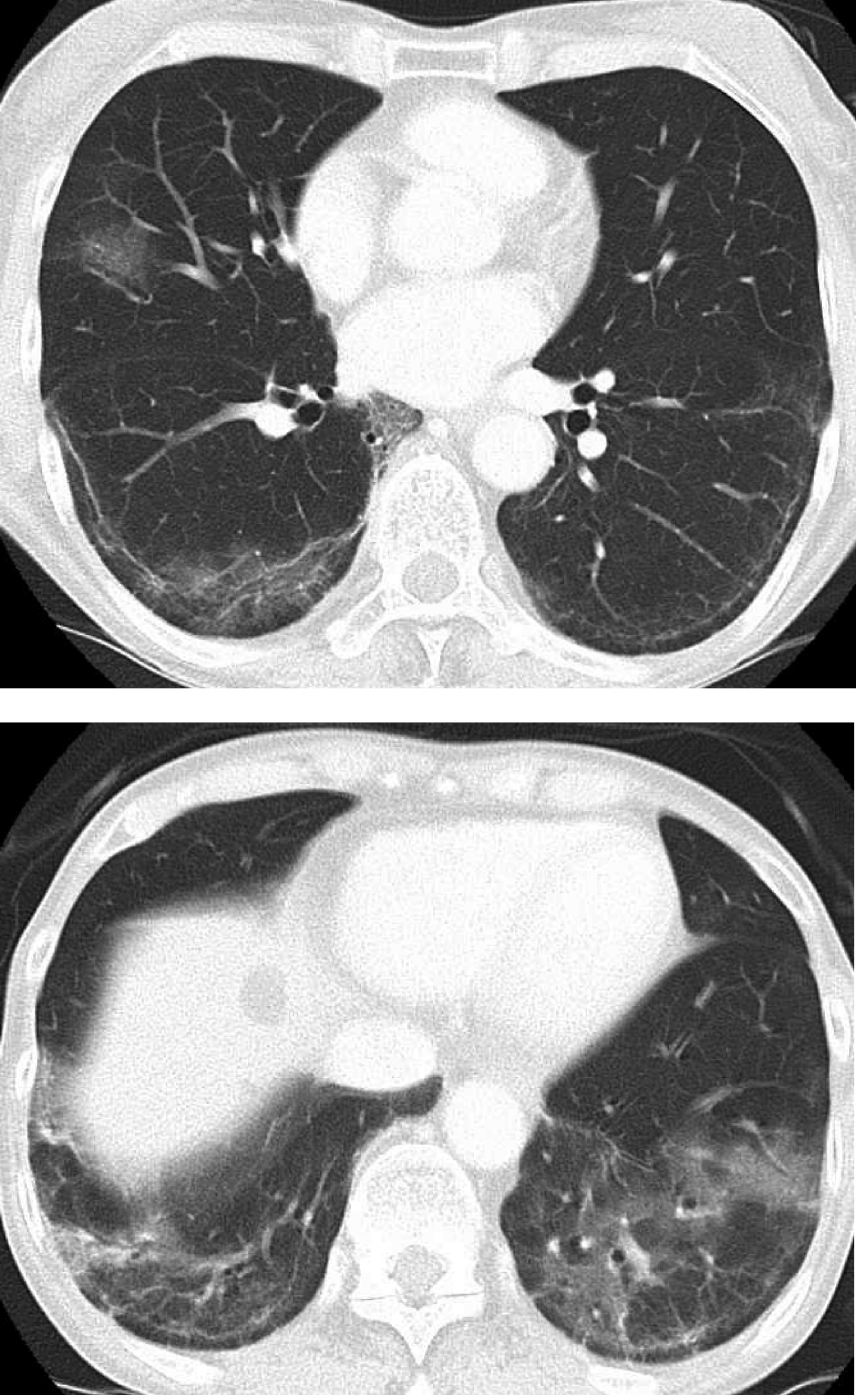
Computed tomography after 2 months from the onset.
